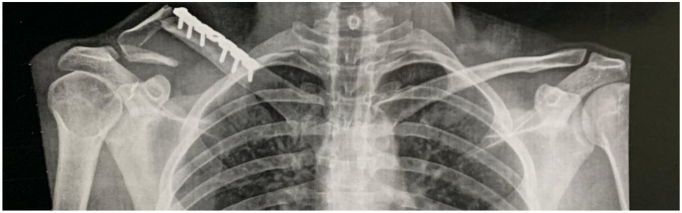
Initial radiographic exam with PIF of the distal third of the clavicle.
